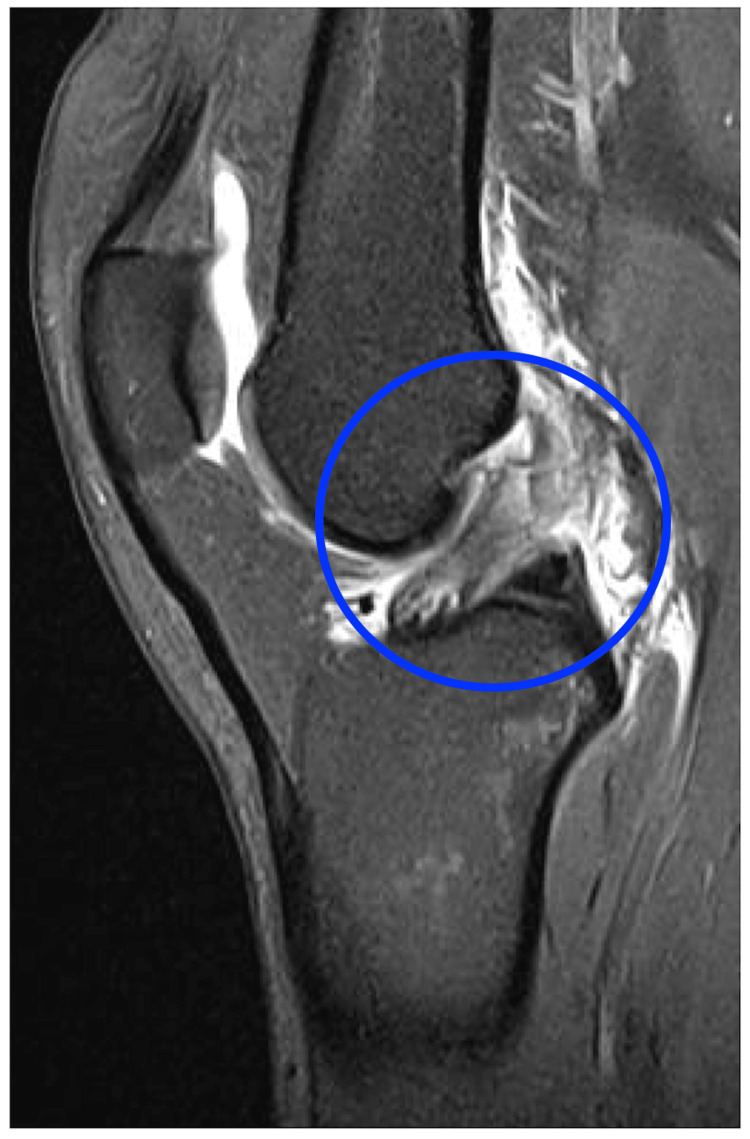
Preoperative MRI (sagittal view) of the right knee demonstrating a proximal anterior cruciate ligament rupture (blue circle).
